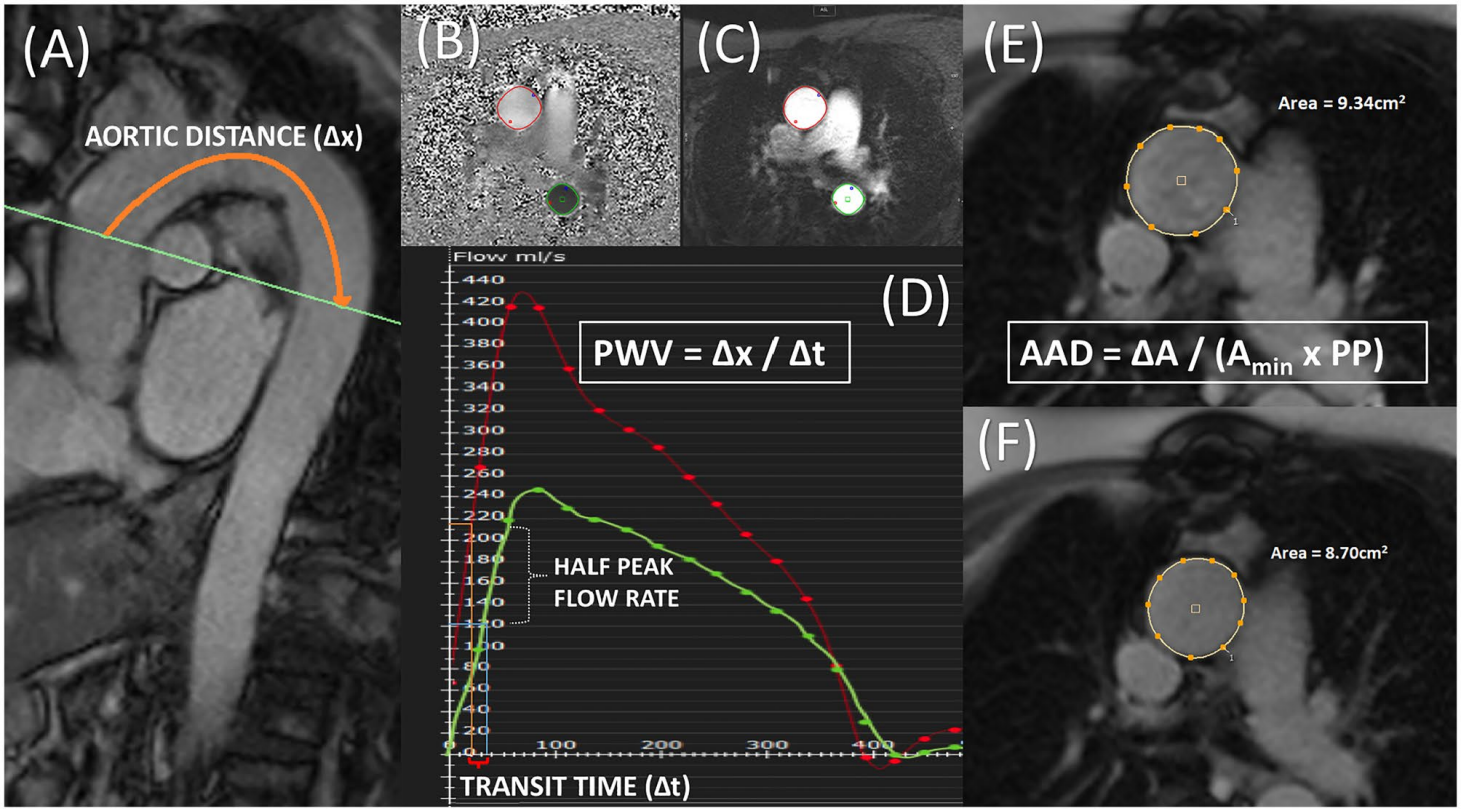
Cardiac magnetic resonance imaging (CMR) measures of arterial stiffness. a–d Pulse wave velocity (PWV) is calculated between the ascending and descending aorta by the transit-time method. A Aortic ‘candy-stick’ cine is used to plan a through-plane velocity flow map, demonstrated by the green line bisecting the pulmonary artery. The aortic distance between the two aortic locations is measured (∆x). b, c Through-plane flow and magnitude images generated from the aortic candy stick. The ascending aorta is contoured in Red and the descending aorta in Green. d Graph demonstrating flow in the ascending aorta (Red) and descending aorta (Green) during systole. Time to half the peak flow rate is measured and the difference calculated as the transit time (∆*t*). PWV is calculated by the equation PWV = ∆*x*/∆*t*. e, f Through-plane cine image of the ascending aorta. The maximal (e) and minimal (f) aortic areas through the cardiac cycle are calculated. Ascending aortic distensibility (AAD) is calculated by the equation AAD = ∆*A* / (*A*_min_ × PP), where ∆*A* represents the change in aortic area, *A*_min_ the minimal aortic area, and PP the pulse pressure
